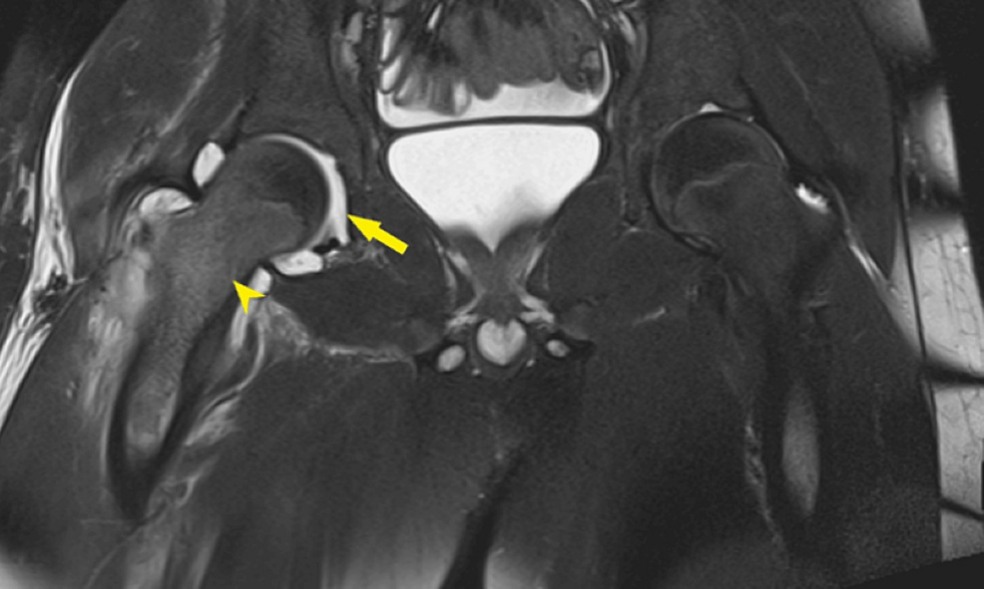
T2-weighted MRI image in the coronal plane shows mild right hip joint effusion (arrow)and bone marrow edema of the femoral neck and proximal shaft (arrowhead)
